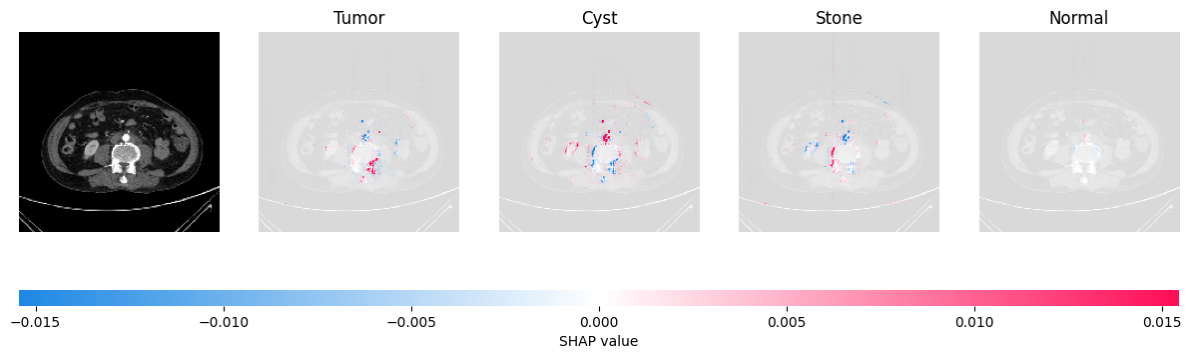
The model’s prediction that the CT image is a cyst is supported by the high concentration of red pixels in the explanation image in the third column.
